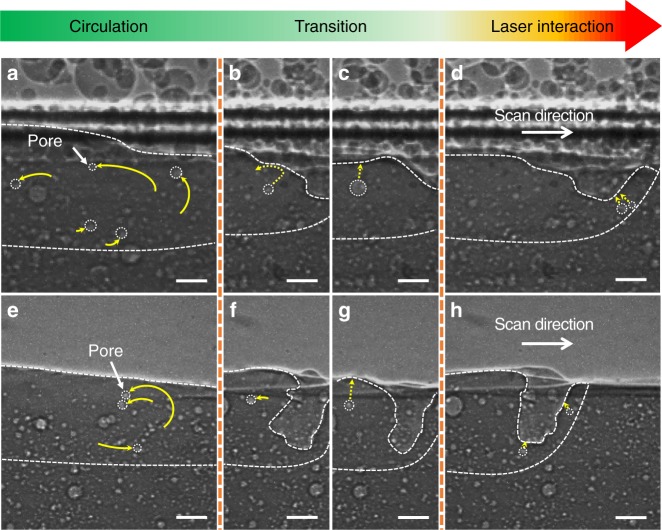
Dynamic pore motions within melt pool. a–d X-ray images showing pore dynamics during the LPBF process. The thickness of powder layer is 100 μm. e–h X-ray images showing pore dynamics during laser melting of a bare substrate. Dotted arrows indicate the future trajectories of the pores, while solid arrows mark the history of pore trajectories. Pores follow circular patterns at the circulation domain (a, e), while pores in the laser interaction domain move toward depression zone and escape from the melt pool (d, h). In the transition domain (b, c, f and g), pores exhibit irregular moving behavior, sometimes moving toward the melt pool surface and escaping (c, g), and sometimes circulating in the melt pool (b, f). The laser beam diameter (*D*4*σ*) is 100 μm, the laser power is 360 W, and the scan speed is 1 m s^−1^. All scale bars are 50 μm
